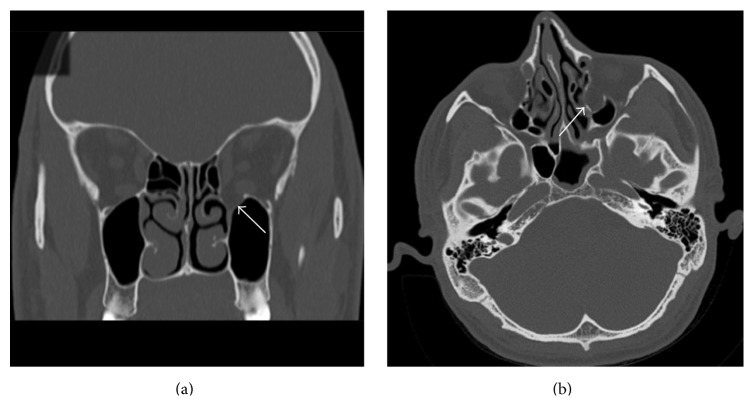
Coronal (a) and axial (b) CT scans showing the old dislocated fracture of the left medial orbital floor and wall.
